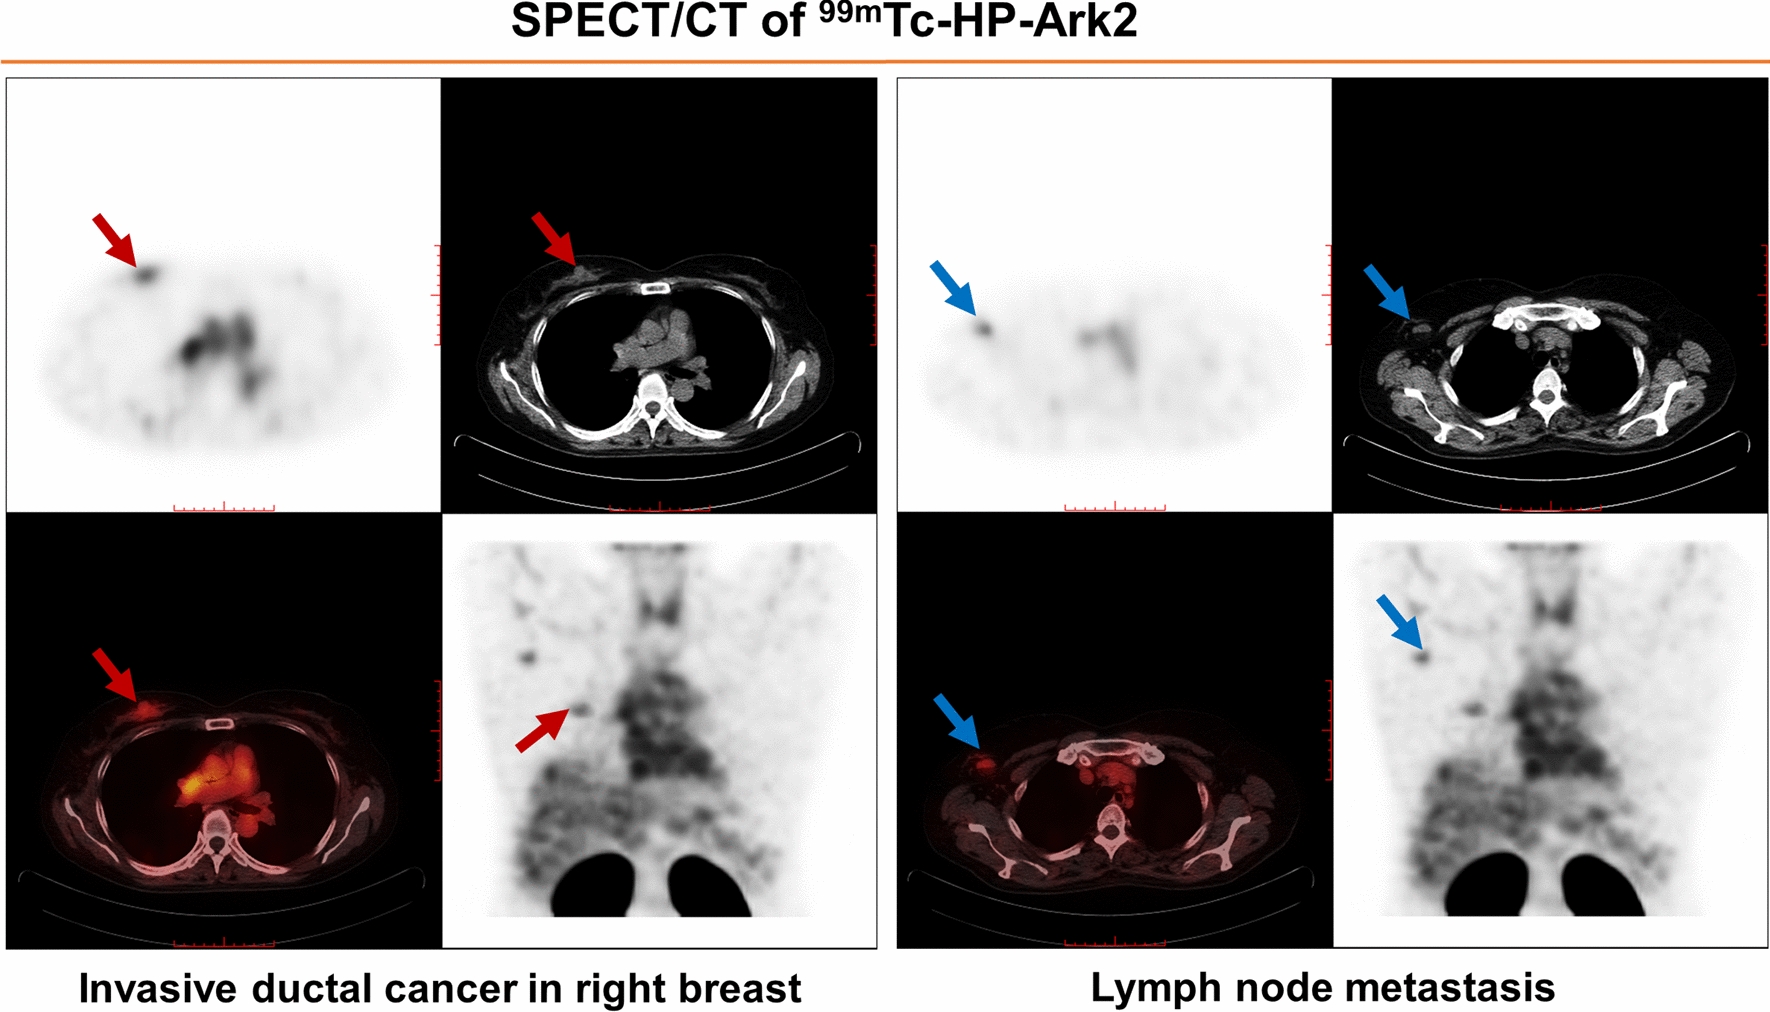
Representative SPECT/CT images of a 58-y-old woman (Patient No. 29) with invasive ductal cancer in her right breast and right axillary lymph node metastasis. The transverse plane SPECT, CT, SPECT/CT fusion and SPECT maximal intensity projection (MIP) images showed intense ^99m^Tc-HP-Ark2 uptake in both the breast tumor (red arrow) and axillary lymph node metastasis (blue arrows)
